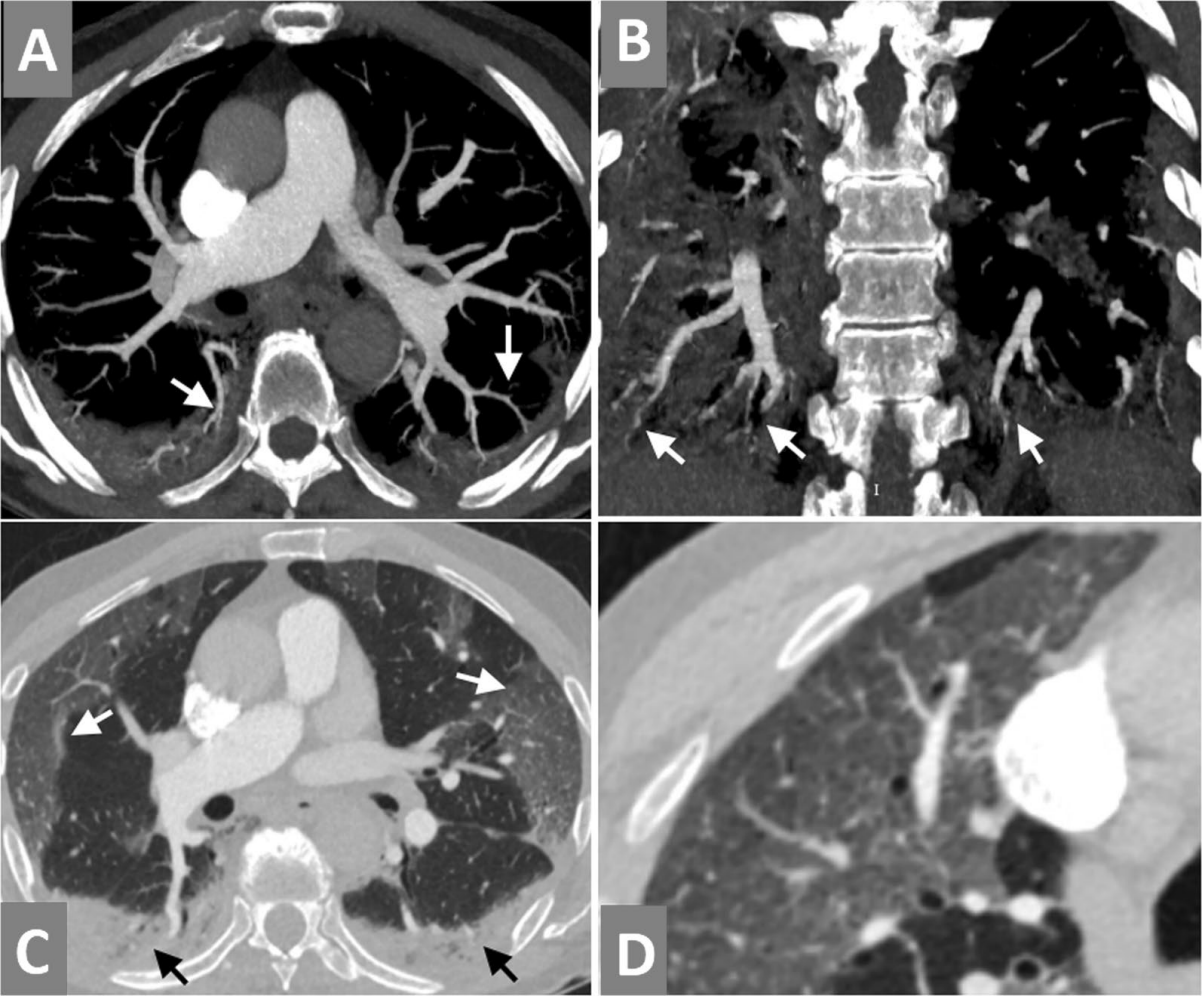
CT-pulmonary angiography (CTPA) examination for a 56-years-old COVID-19 male patient in the second week of infection. [A] Axial CTPA and [B] Coronal CTPA images showed bilateral basal dilatation of the peripheral pulmonary arterial tree reaching the pleural surface with a "loss of normal tapering" sign. [C] Corresponding axial CT image (lung window) showed bilateral sub-pleural ground-glass patches with exaggerated density at the basal gravitational lung zones. [D] Zoomed axial CT image (lung window) showed asymmetrical abnormal dilatation of pulmonary arterial branch inside the right upper lobar ground-glass patch … Pulmonary vascular enlargement (an indirect sign of pulmonary vasculopathy)
